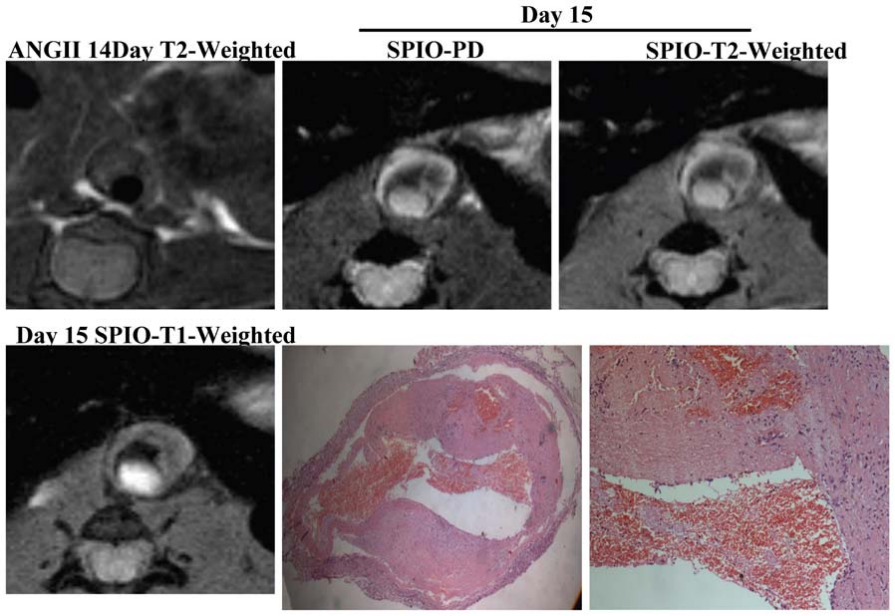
Acute thrombus formation. Compared with 14 days of ANG II infusion, MRI at day 15 showed acute artery occlusive and mural thrombi (red arrow hyperintense on T1-weighted images). HE showed fresh thrombus with platelets, inflammatory cells, and fibrin mesh.
